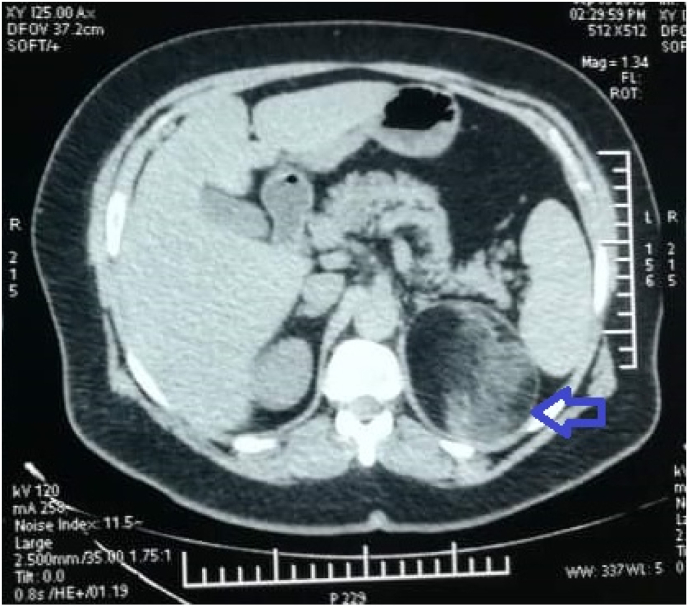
Abdominal computed tomography scan showing a large well-defined mass of the left adrenal gland with fat density suggesting myelolipoma (Blue arrow).
